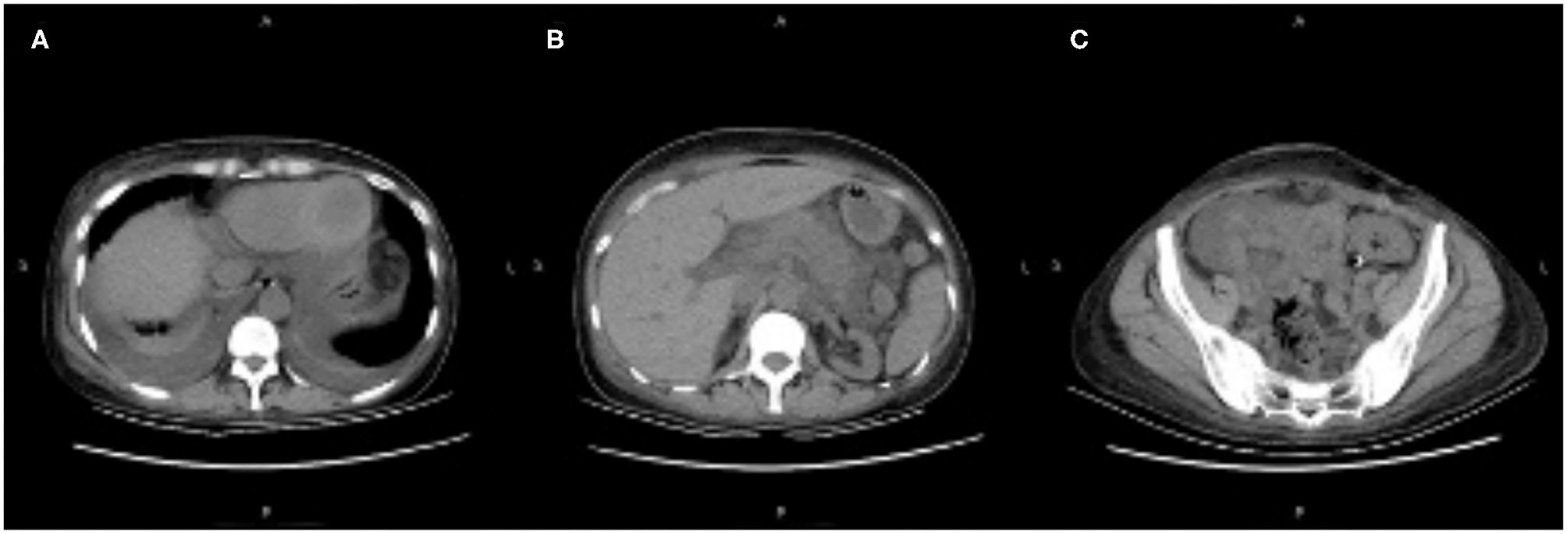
(A–C) Abdominal computed tomography scan showing pancreatic enlargement with peripancreatic inflammatory exudate, bilateral pleural effusion, and the transplanted kidney.
